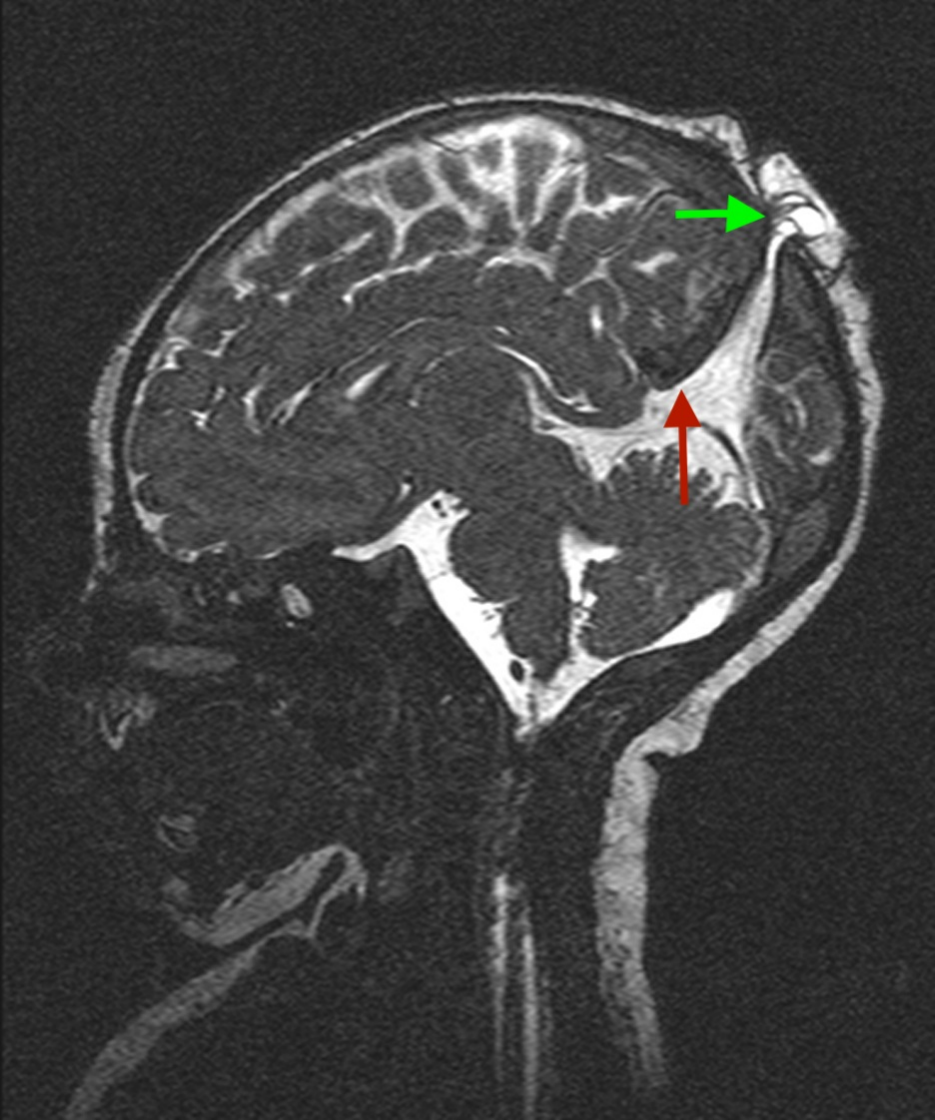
Sagittal CISS T2-enhanced brain MRI showing encephalomeningocele and associated membranes (green arrow), and persistent fetal vein (red arrow) CISS: constructive interference in steady state; MRI: magnetic resonance imaging.
